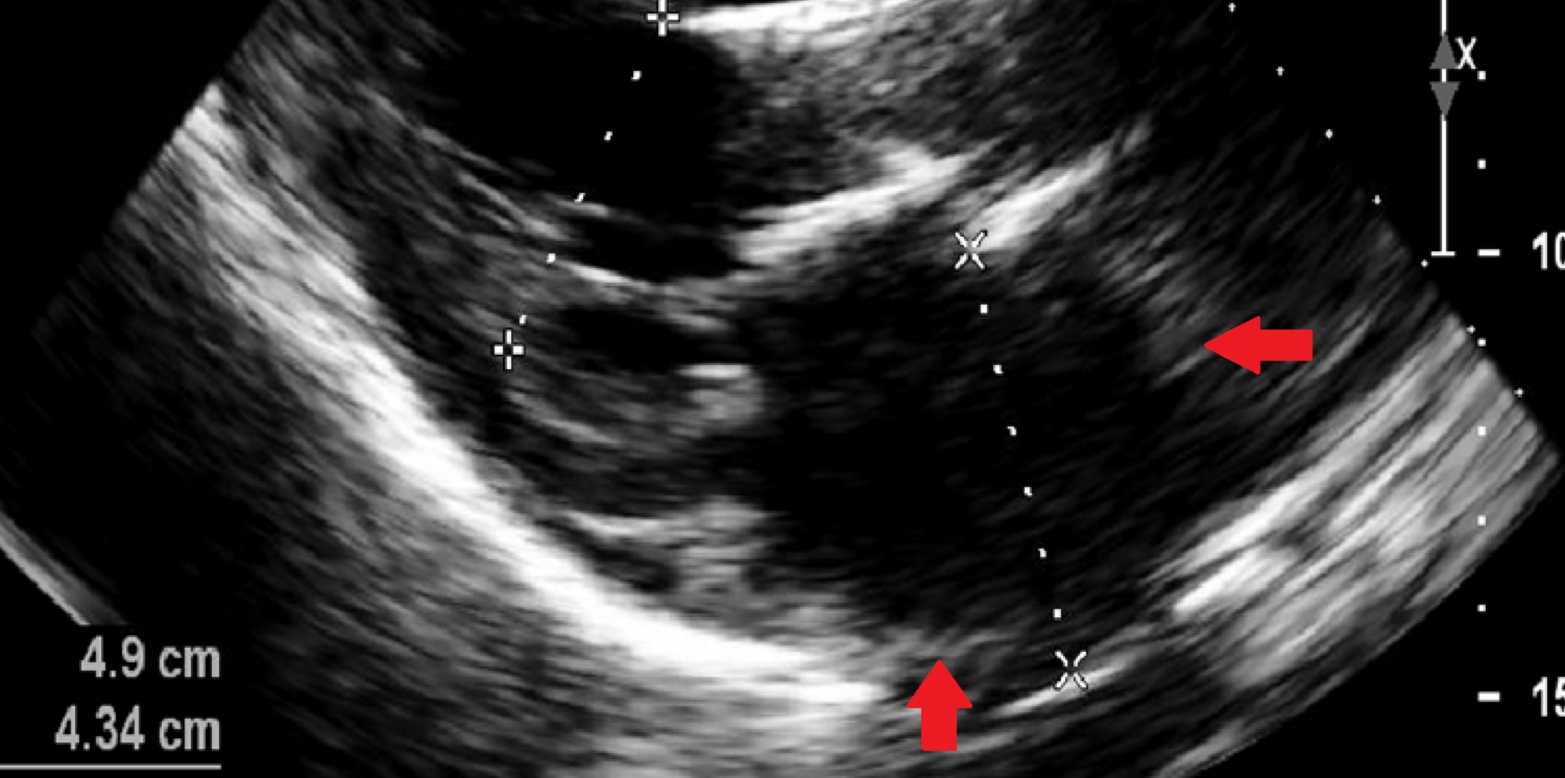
Transthoracic echocardiogram with a parasternal long-axis view showing dilated left atrium
